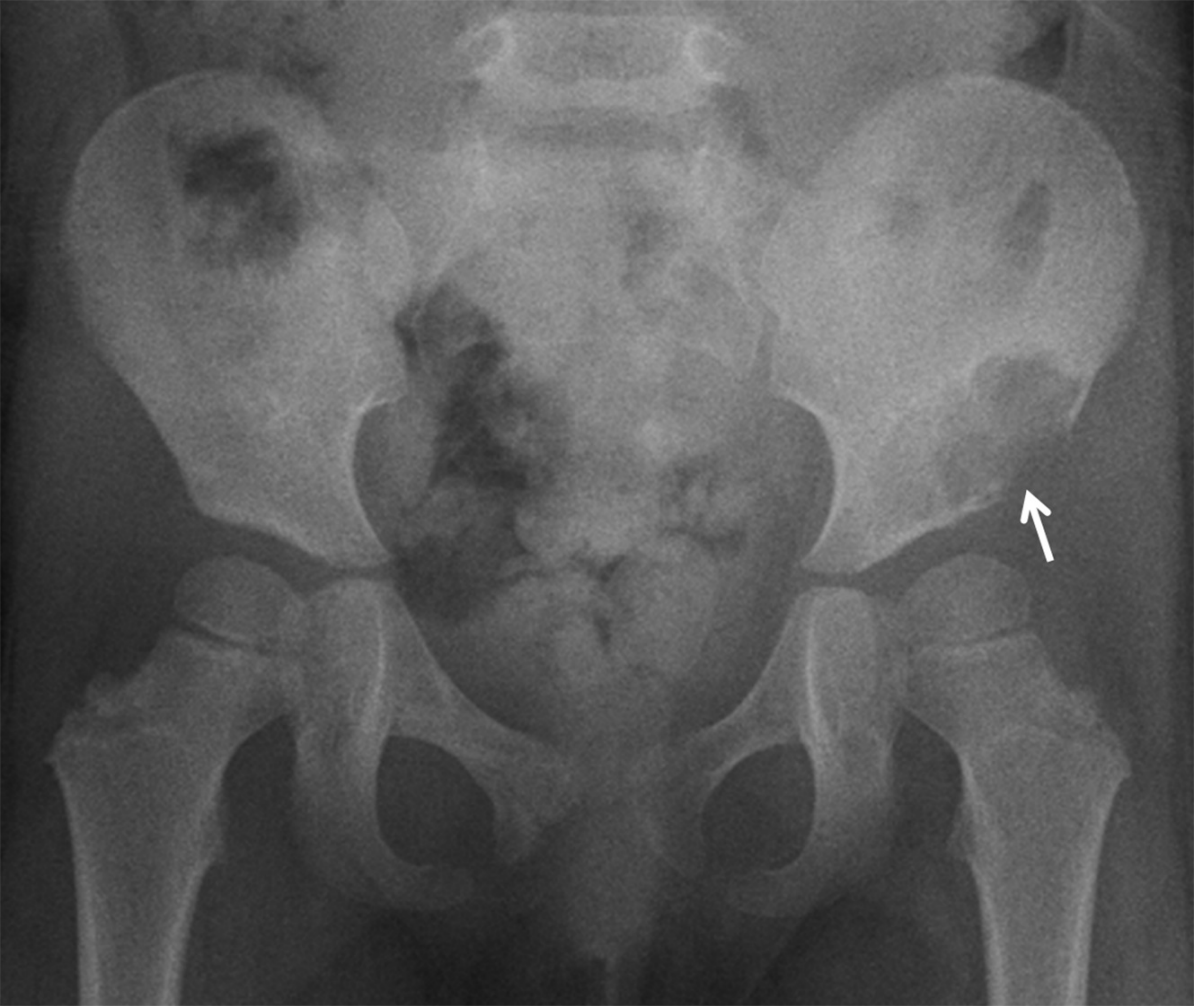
Plain radiograph of the pelvis demonstrates an osteolytic lesion in the left iliac wing (arrow).
